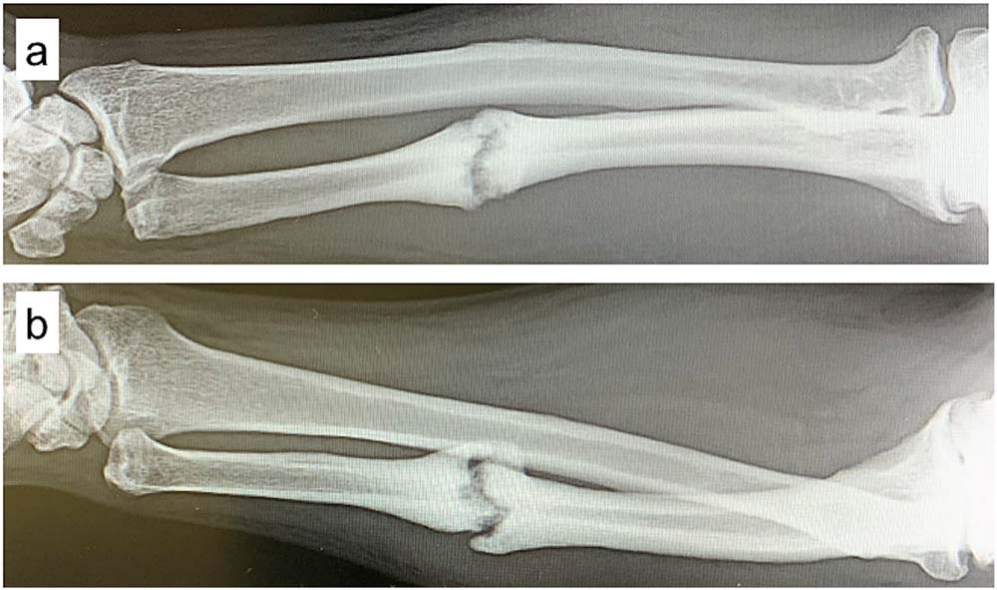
Plain radiographs at initial presentation. (a, b) Nonunion at the mid-diaphysis of the ulna is observed.
